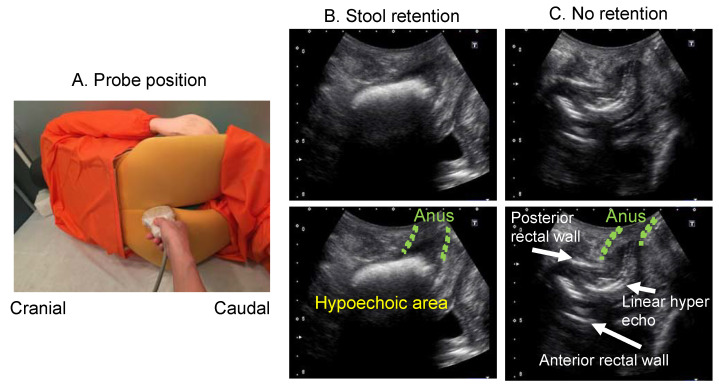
Probe position and rectal ultrasound images in intergluteal cleft scanning method. (A) Probe position. By placing the subject in a side-lying position and flexing the knees, a space is created between the coccyx and the anus. The probe is applied to this area with a longitudinal scan. (B) US image when there is stool retention. The stool in the lower rectum is observed as a hyperechoic area. (C) Ultrasound image when there is no stool retention. When stool is not accumulated, a hyperechoic line is observed, and even the anterior rectal wall is observed.
